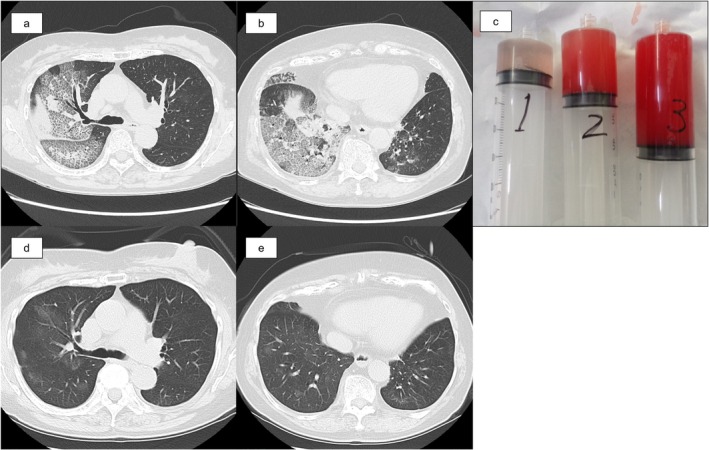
Test results of Patient B. Chest CT on the day of admission. Infiltrative shadows with ground‐glass opacities and interlobular septal thickening were observed in the right upper and lower lobes (a), and they were also present in the right lung middle lobe (b). Small areas of ground‐glass opacity were also observed in the lower lobe of the left lung (b). The patient's BALF appeared progressively bloodier. The three syringes, arranged from left to right, represent the first, second, and third BALF collections, respectively (c). CT at 1‐month post‐discharge. Bilateral upper and lower lobe infiltrates have almost resolved (d, e).
